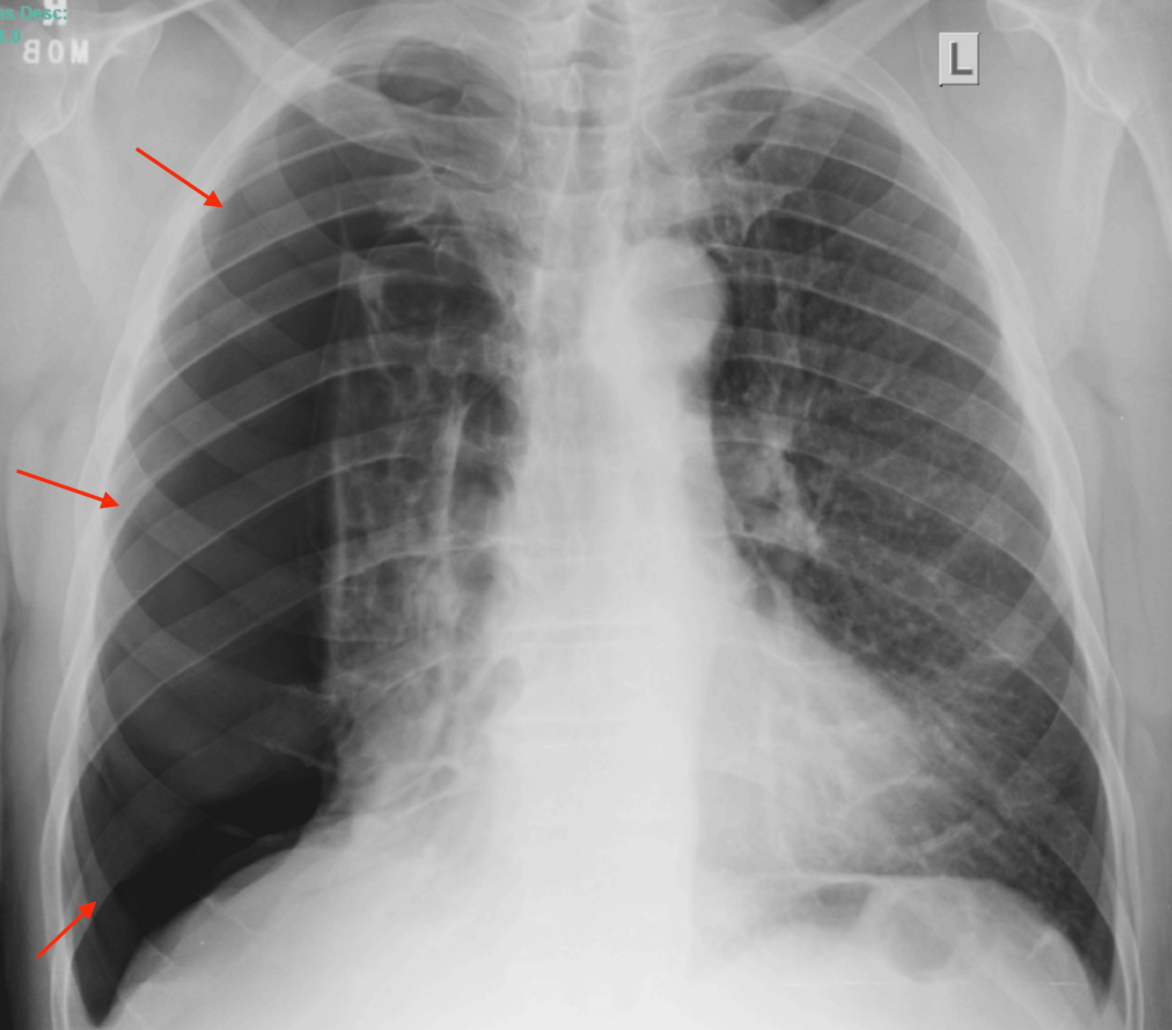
Pre-intervention chest radiograph showing large right-sided pneumothorax.
